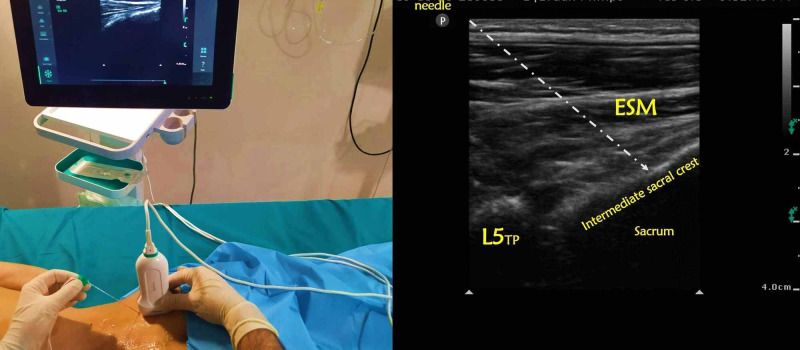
Patient and ultrasound setup for sacral erector spinae plane block; sonographic anatomy of the block L5: lumbar 5th vertebrae; ESM: erector spinae muscle
